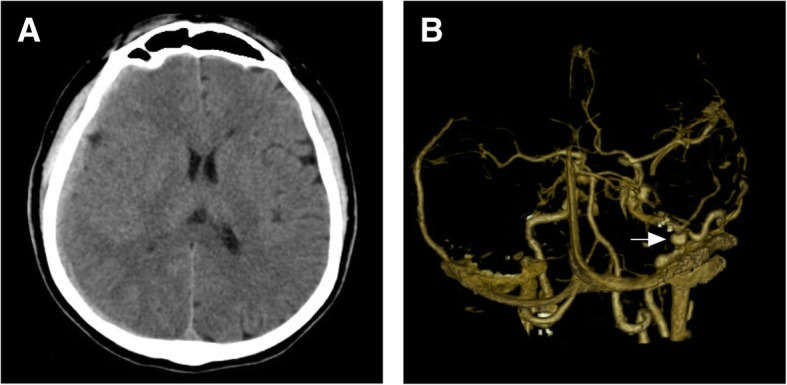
(a) Head CT shows an isodense CSDH on the right hemisphere with mild midline shift. (b) CTA reveals a DAVF located at the transverse sinus with dilated cortical venous drainage (arrow). CT: computed tomography; CSDH: chronic subdural hematoma; CTA: CT angiography; DAVF: dural arteriovenous fistula
